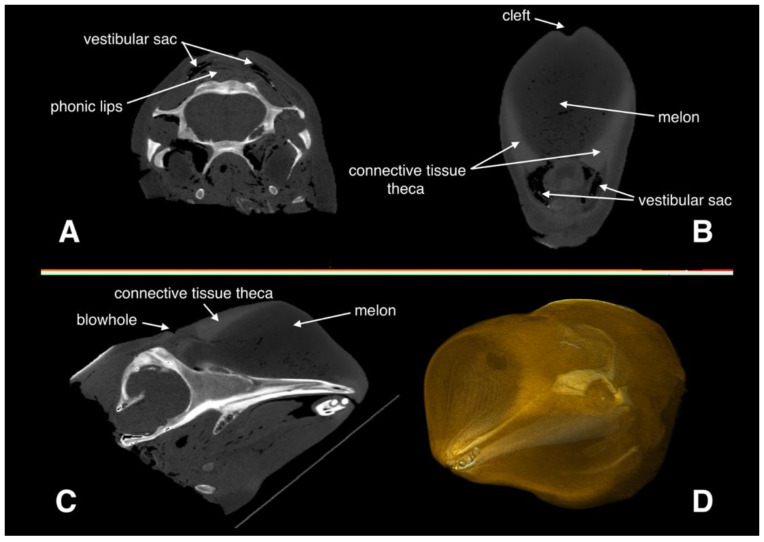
CT scan of the Risso’s dolphin’s head. (A) Cross plane, (B) frontal plane, and (C) sagittal plane of the head. The gray level represents the different HU values. (D) 3D reconstruction of the head. The yellow transparent parts are soft tissue.
